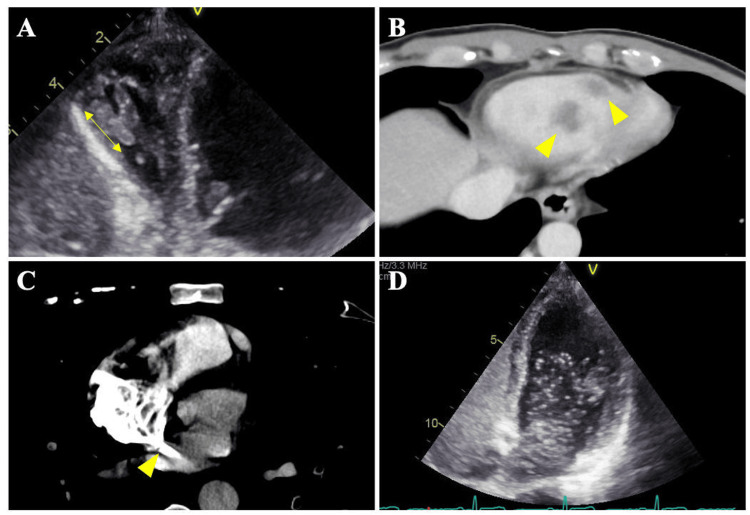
Cardiac imaging (A) Point-of-care ultrasound showed a mobile right ventricular thrombus (RVT) with a length of 15 mm. (B) Chest contrast-enhanced computed tomography (CT) revealed multiple RVT (yellow arrowhead). (C) Chest contrast-enhanced CT revealed contrast agent flow from the right atrium to the left atrium, suggesting a shunt through a patent foramen ovale (PFO) (yellow arrowhead). (D) Transthoracic echocardiography showed multiple bubbles in the left atrium and ventricle through the PFO.
